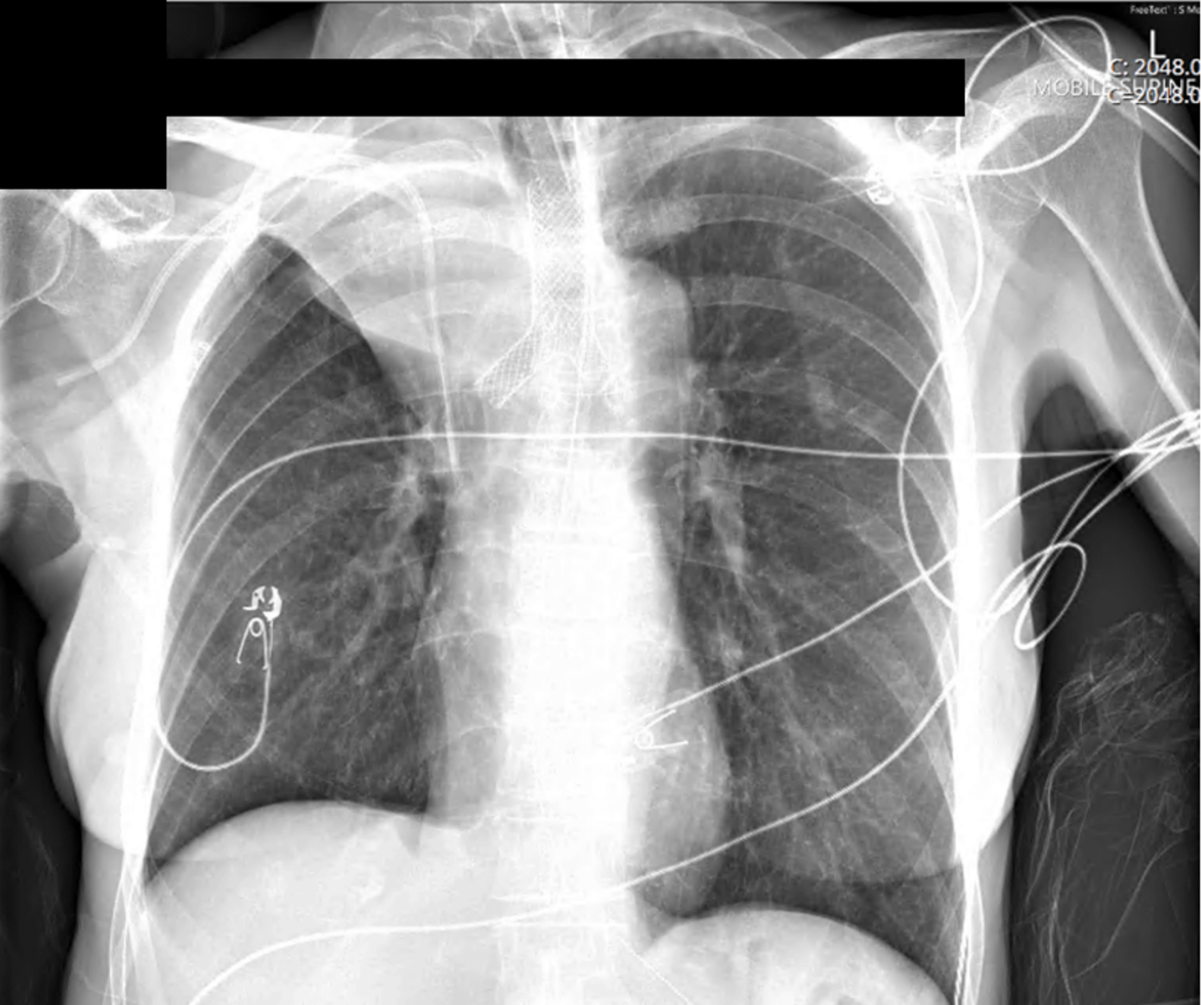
Postoperative AP CXR demonstrating reversed nitinol-coated Y stent (note: position with long left main bronchus projection intentionally deployed to the RMB) with sacrifice of the right upper lobe (RUL), but restoration of right middle lobe (RML) and right lower lobe (RLL) aeration. AP CXR, anteroposterior chest X-ray; RMB, right main bronchus
